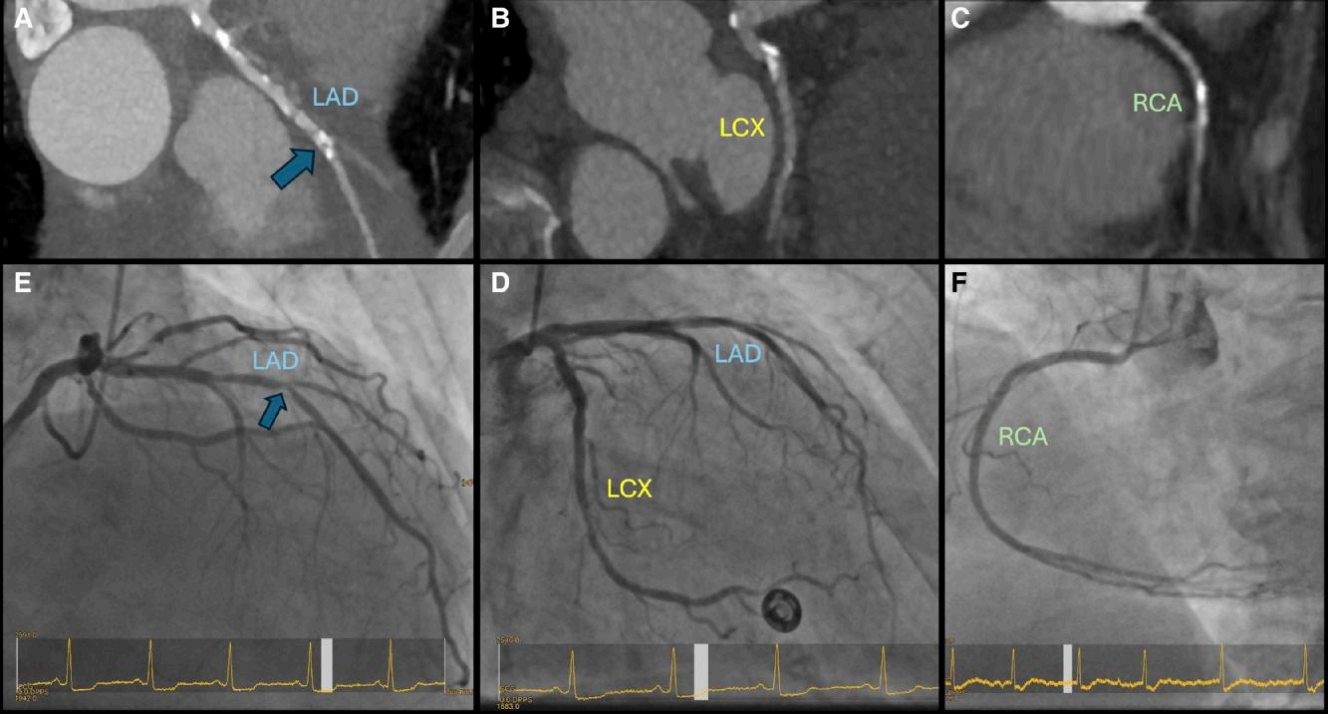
CTA (TAVI planning CT) and corresponding invasive CA and significant coronary disease. A 86-year-old male patient, dyspnoea on exertion NYHA III, no angina pectoris, and impaired kidney function. High-gradient AS, peak velocity on echocardiography 4.1 m/s. *A* shows left main stem and LAD with significant calcification and stenosis (blue arrow), *B* shows the LCX, which is also calcified, and *C* shows the RCA, all from the TAVI planning CT. *D–F* depict the corresponding images of the coronaries by invasive CA. The LAD was significantly stenosed. The patient underwent elective PCI of the LAD prior TAVI. CT, computed tomography; LAD, left anterior descending artery; LCX, circumflex artery; RCA, right coronary artery; TAVI, transcatheter aortic valve implantation.
